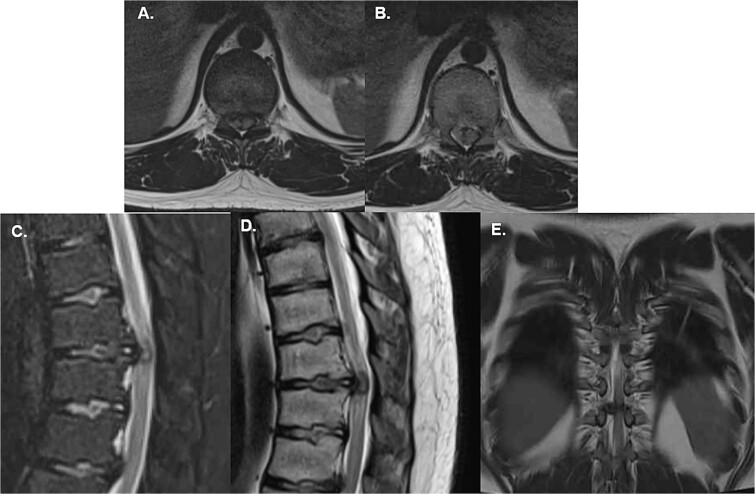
Emergency MRI of the patient shows (A) and (B) axial T2W projections that demonstrate a severe compression of the spinal cord given the central location of the extruded disc, (C) a sagittal T2W with fat suppression allows to see the extruded disc nucleus of the T10-T11 disc causing compression of the spinal cord; edematous changes are also visible, (D) shows a T1W sagittal midline image of the extruded disc which also shows a flattened spinal cord with associated edema; note the absence of any calcification or changes associated with chronic progression and (E) is a coronal T2W image of the disc herniation showing its central location with a slight predominance over the right canal.
